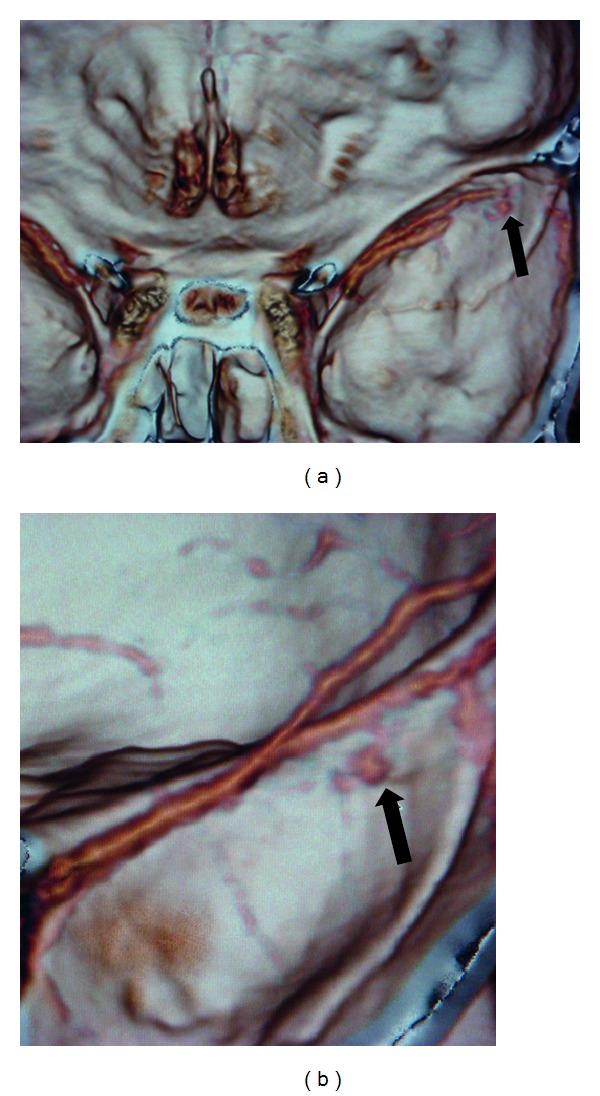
(a) Computed tomography angiography that displayed middle meningeal artery pseudoaneurysm. (b) More detailed image of the vascular lesion in computed tomography angiography with three-dimensional reconstruction of vessel and middle cranial fossa.
